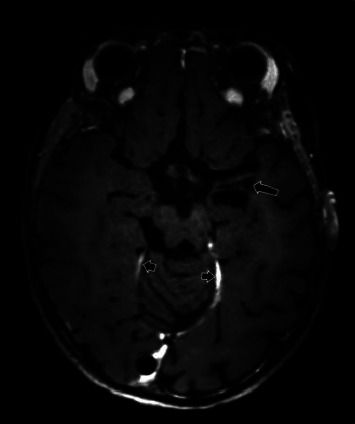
Follow-up MRI 18 months later. Vessel wall imaging (axial 3D T1 TSE SPACE Dante) after IV gadolinium administration. The diffuse leptomeningeal contrast enhancement and vessel wall contrast enhancement are no longer visible. There is limited residual contrast enhancement of the cerebellar tentorium (short arrow), and focal cystic encefalomalacia in the left mesial temporal lobe (long arrow) and basal ganglia (not shown) after ischemic infarction.
